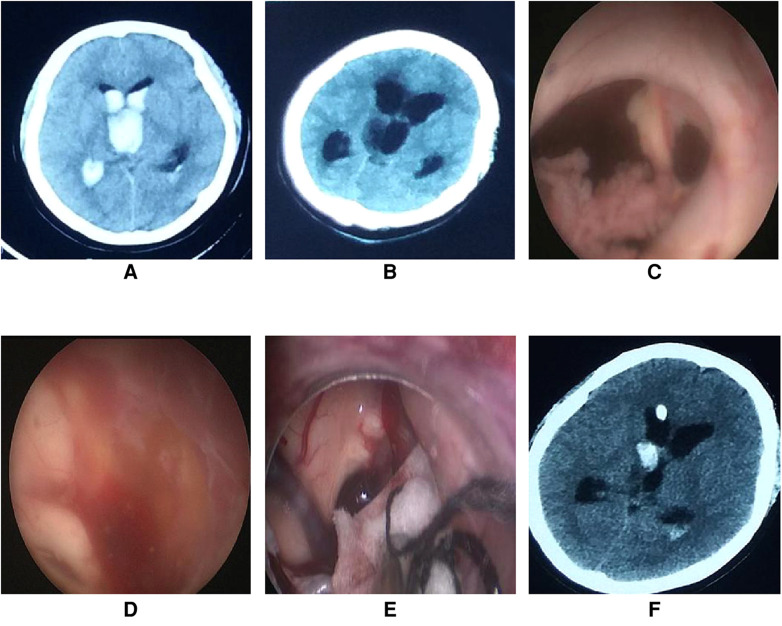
Endoscopic intraventricular surgery was performed to perform ETV in case 14. (A) On admission, CT scans revealed a ventricular hemorrhage with hydrocephalus. (B) CT scan of hydrocephalus on the third day after extubation of the drainage catheter. (C) Connective tissue was found in the foramen of Monro during surgery. (D) The third ventricle was full of red-yellow hemosiderin and the bottom of the third ventricle was unrecognizable. (E) A patty and slim bipolar was used to stop the bleeding through the extra-endoscopic technique. The source of bleeding was located in the choroid plexus of the lateral ventricle near the foramen of Monro. (F) CT scans on the first day after endoscopic surgery showed a small hemorrhage in the right lateral ventricle.
